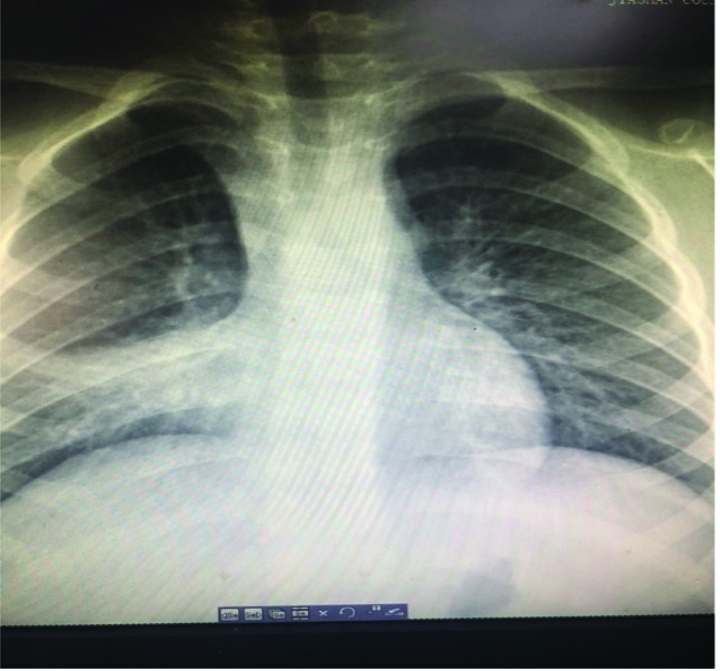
X-ray showing laminar atelectasis in the right middle lobe.
